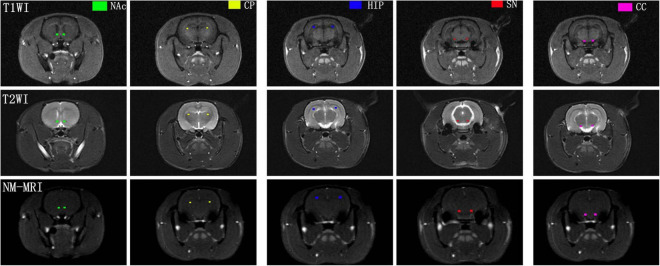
Representative NM-MRI images of brain regions of interest. CP, caudate putamen; NAc, nucleus accumbens; HIP, hippocampus; SN, substantia nigra; CC, crus cerebri.
